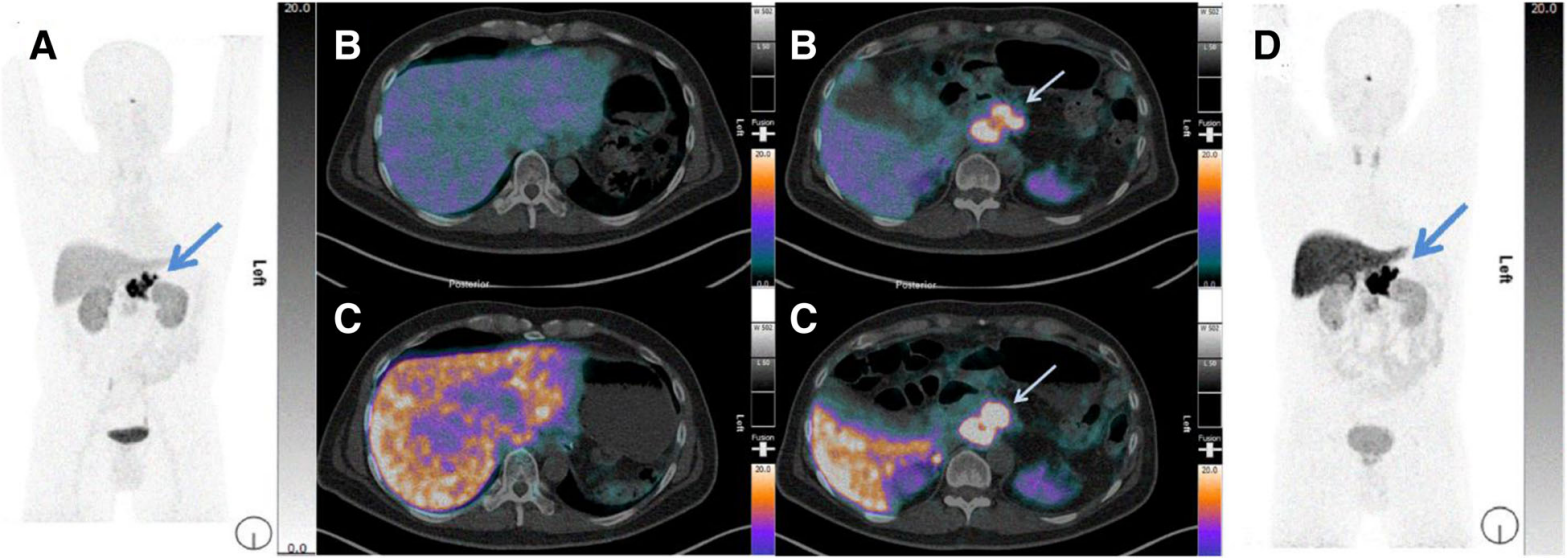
[^68^Ga]Ga-DATA-TOC and [^68^Ga] Ga-DOTATOC PET/CT images. a [^68^Ga]Ga-DATA-TOC PET MIP. b Transverse PET/CT fusion of [^68^Ga]Ga-DATA-TOC PET/CT images. c Transverse PET/CT fusion [^68^Ga]Ga-DOTA-TOC PET/CT images. d [^68^Ga]Ga-DOTA-TOC PET MIP. Arrows indicate high uptake in the primary pancreatic NET. The [^68^Ga]Ga-DATA-TOC PET/CT images show slightly higher physiological uptake in the kidneys as compared to the [^68^Ga]Ga-DOTA-TOC PET/CT study in the same patient. There is significantly higher uptake in normal liver after injection of [^68^Ga]Ga-DOTA-TOC in comparison with PET/CT images obtained after using [^68^Ga]Ga-DATA-TOC
